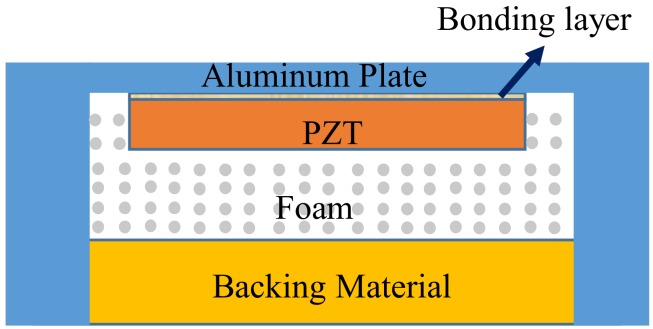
The cross sectional view of a typical ultrasonic sensor.
